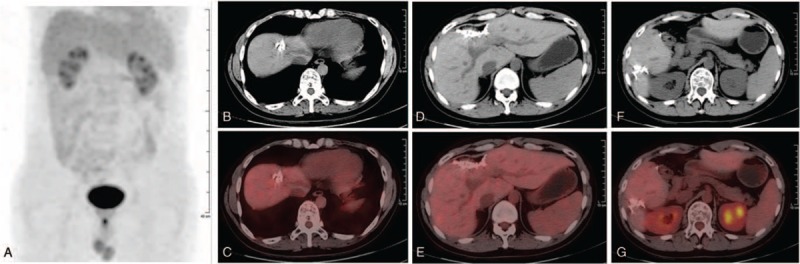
The follow-up ^18^F-FDG PET/CT images 3 yr after treatment. Both the MIP (A) and axial images (CT: B, D, and F; PET/CT fusion: C, E, and G) showed no abnormal uptake in the abdomen. ^18^F-FDG = ^18^F-fluoro-2-deoxy-D-glucose, MIP = maximum intensity projection, PET/CT = positron emission tomography/computed tomography.
